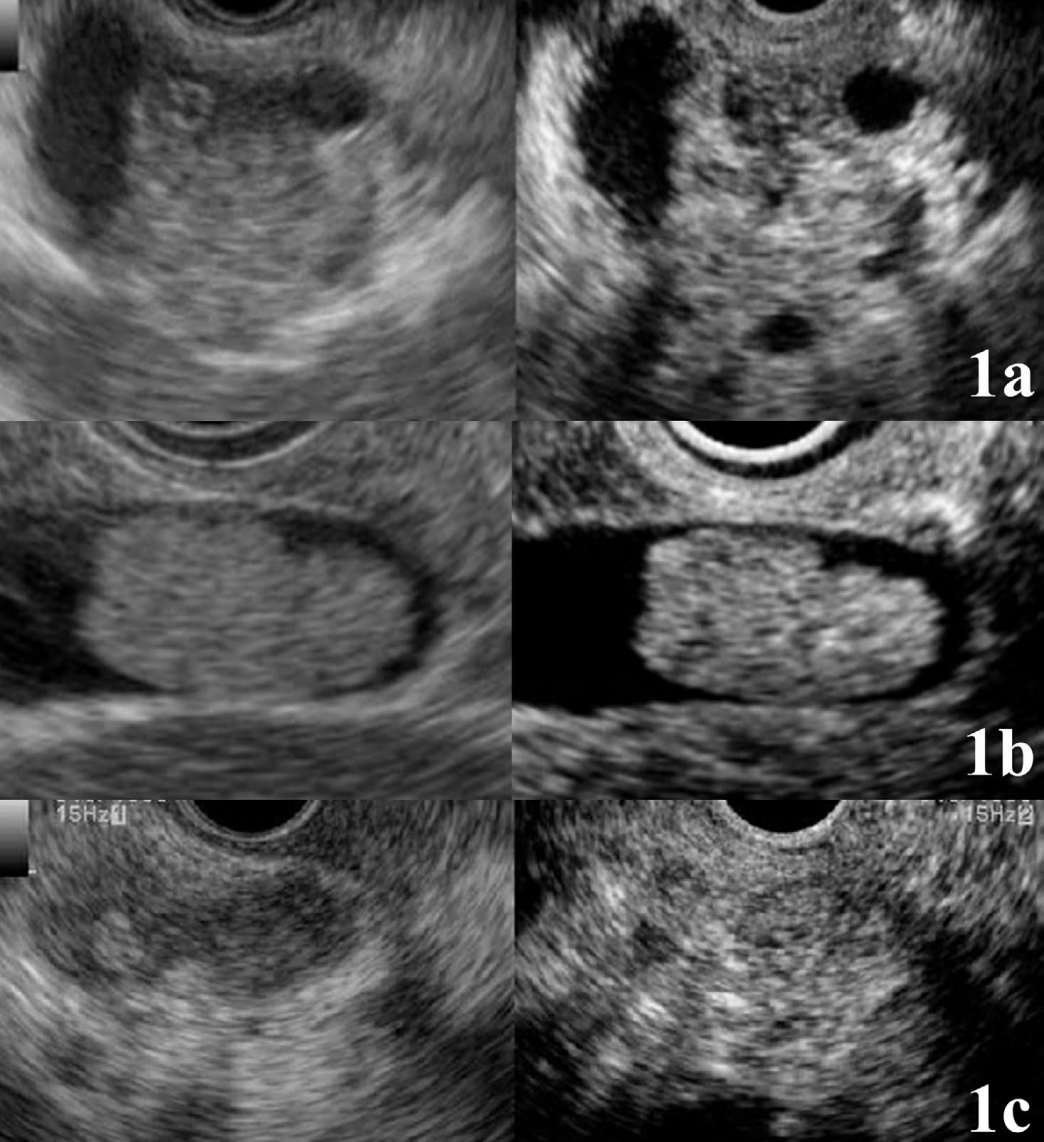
Representative CE‐EUS images showing (a) advanced gallbladder cancer with disrupted wall layer structure (T3), (b) early gallbladder cancer with clear wall layer structure (T1a), and (c) xanthogranulomatous cholecystitis with unclear wall layer structure resulting in a false‐positive interpretation. CE‐EUS, contrast‐enhanced endoscopic ultrasonography.
